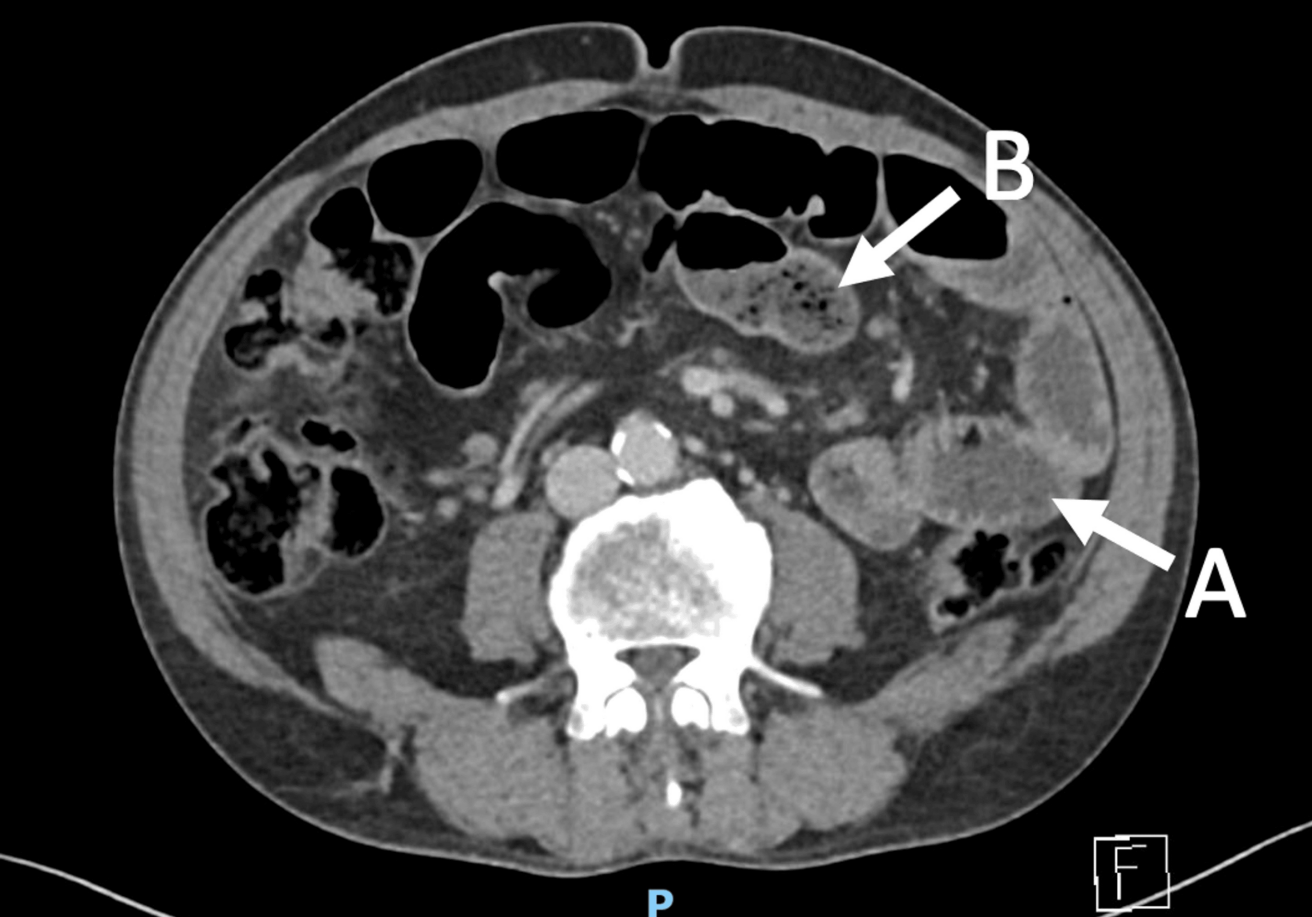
Axial view of CT scan. (A) Dilated small bowel loops and (B) small bowel fecalization and air-fluid levels.
